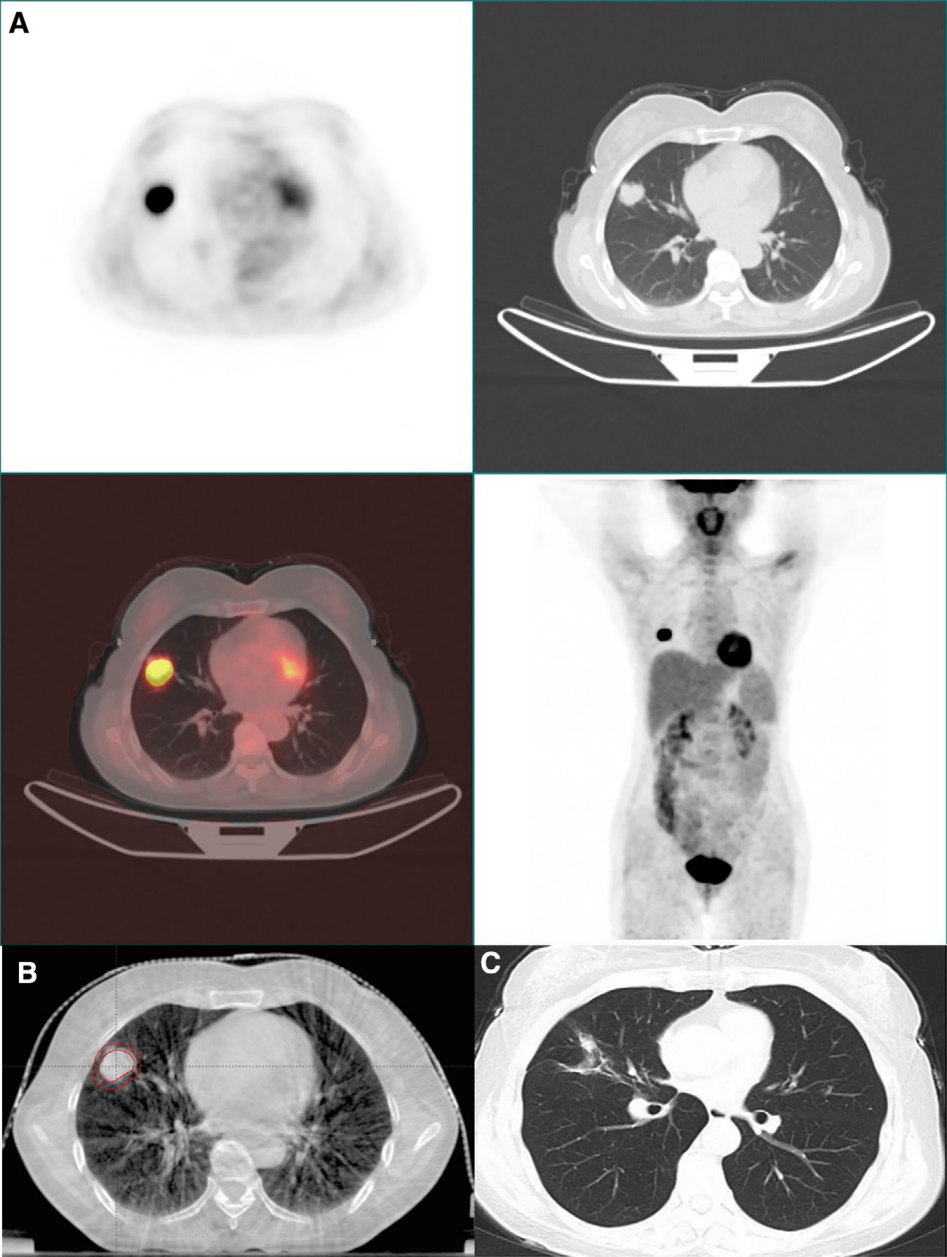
The pretreatment PET/CT images (A), the cone beam CT image before first delivery (B) and the chest CT 40 months after treatment (C) [Color figure can be viewed at http://wileyonlinelibrary.com]
